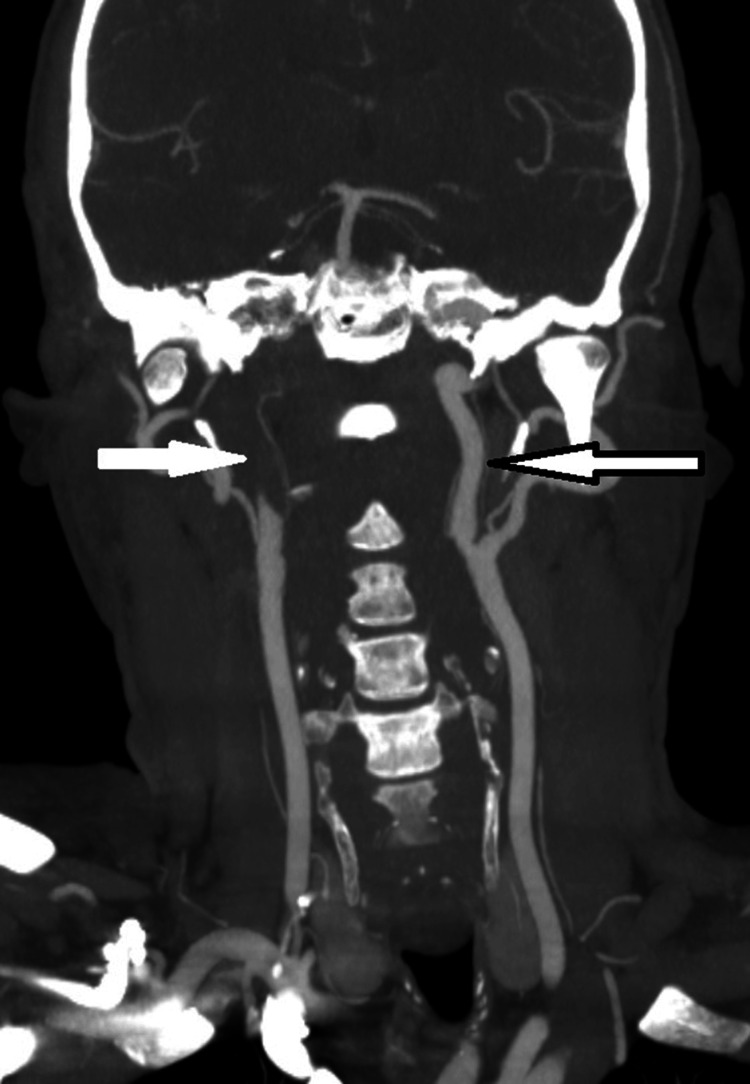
CT angiogram (coronal view) of the aortic arch and carotids, revealing complete occlusion of the right ICA (white arrow), and a patent left ICA (arrow with black borders), approximately 1.5 cm from the bifurcation. CT, Computed Tomography; ICA, Internal Carotid Artery
